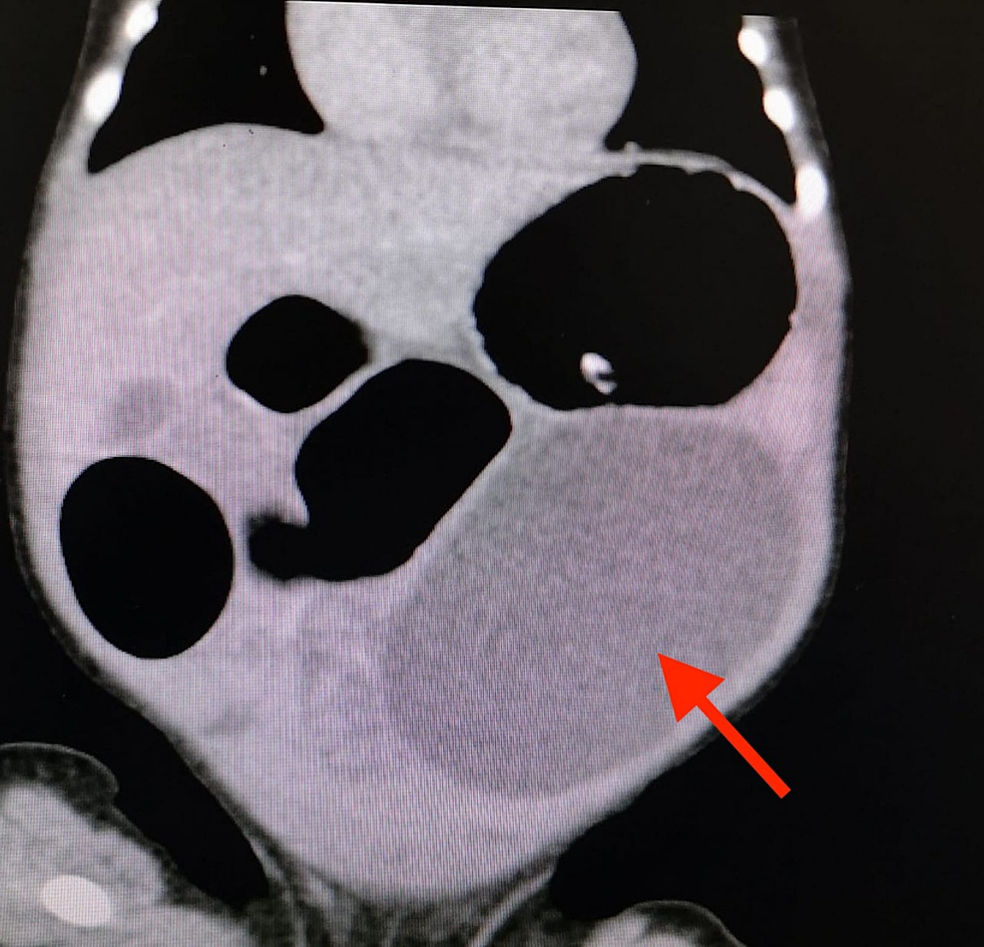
An abdominal CT scan showing a left-sided cystic lesion notably obstructing the small bowel (red arrow). CT, computed tomography
